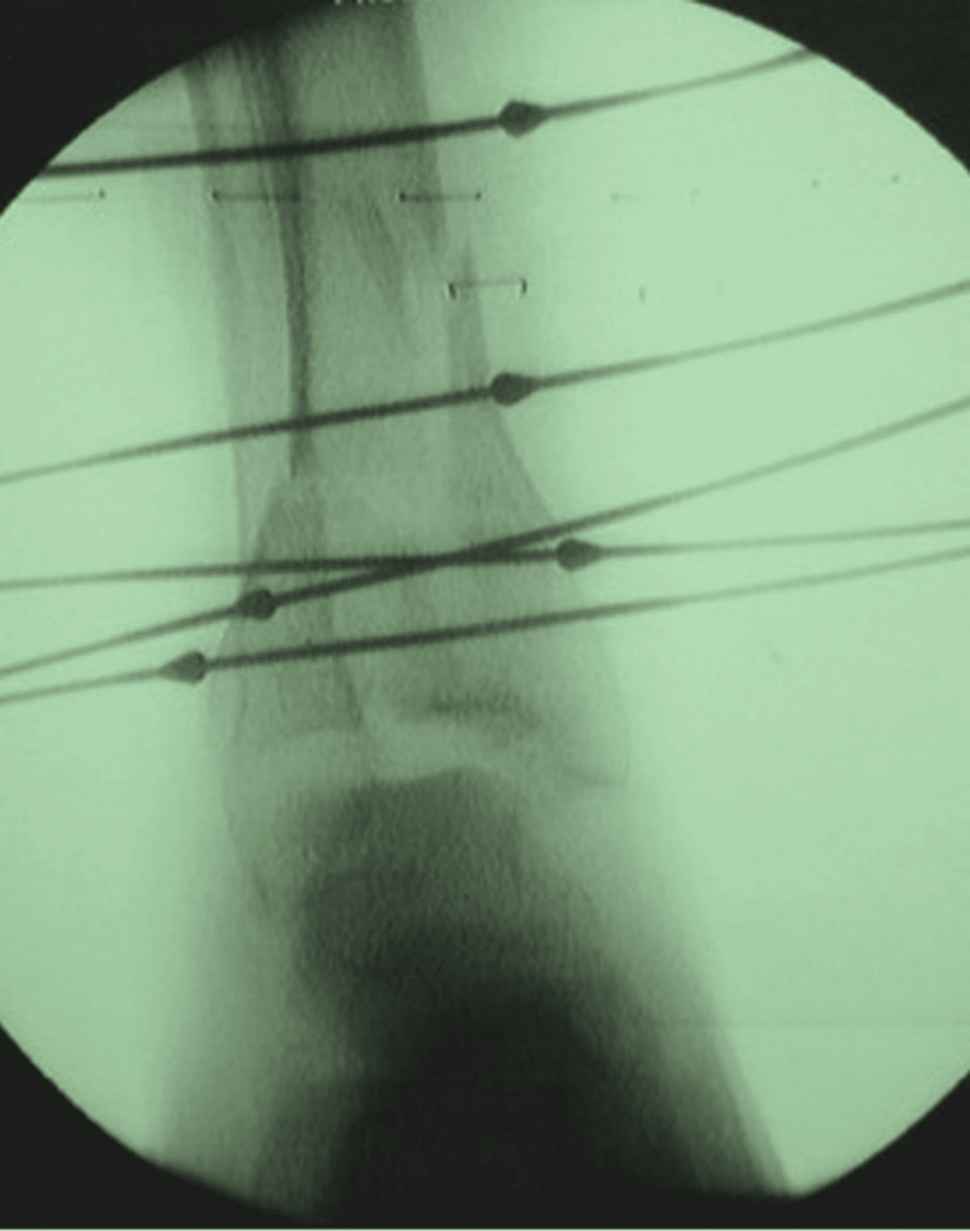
Anteroposterior radiograph of a pilon fracture and its reconstruction using the Ilizarov method
